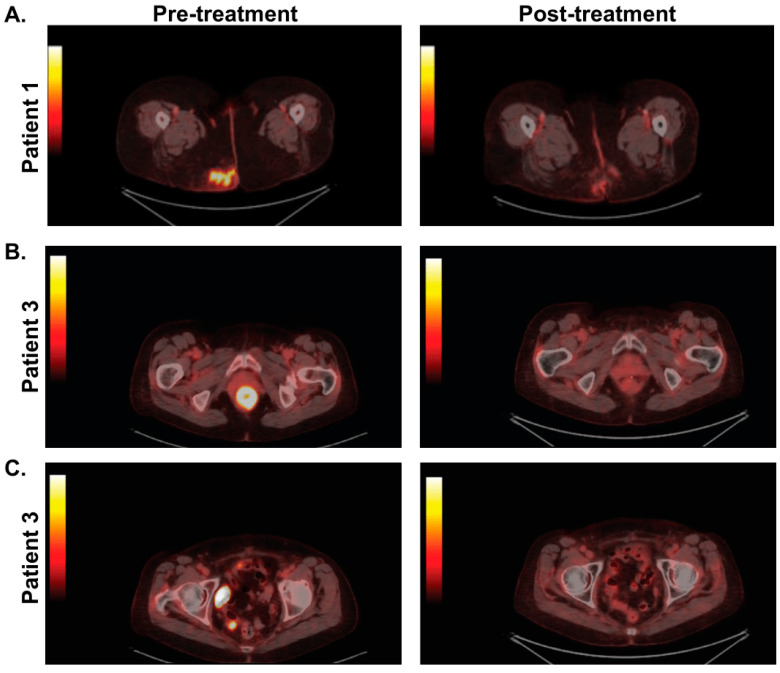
FDG PET/CT imaging pre- and post-treatment with carboplatin and paclitaxel chemoradiation. (A) Patient 1 had a squamous cell perianal cancer with gluteal extension pre-treatment and with resolution of the PET avidity in this area post-treatment. (B) Patient 3 had a highly PET-avid squamous cell carcinoma within the anal canal. (C) This patient also had extension to regional lymph nodes. The PET avidity resolved in both of these areas following chemoradiation.
